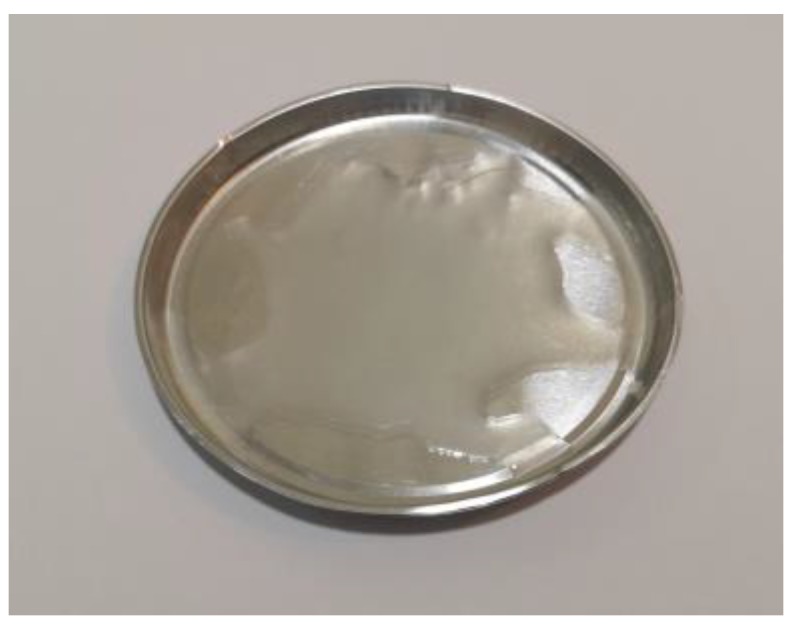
Preparation of epoxy/OMMT thin film for WAXS (Wide-angle X-ray analysis) analysis.
